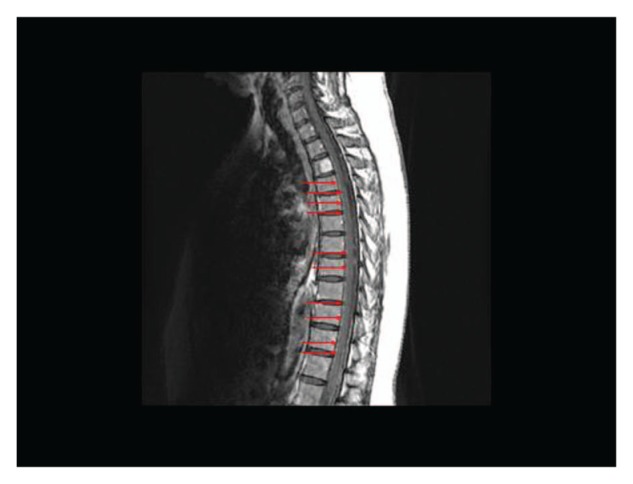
Saggital T1-weighted postcontrast magnetic resonance image of lower cervical and thoracic spine demonstrating subtle diffuse leptomeningeal enhancement of the spinal cord, as indicated by the red arrows.
